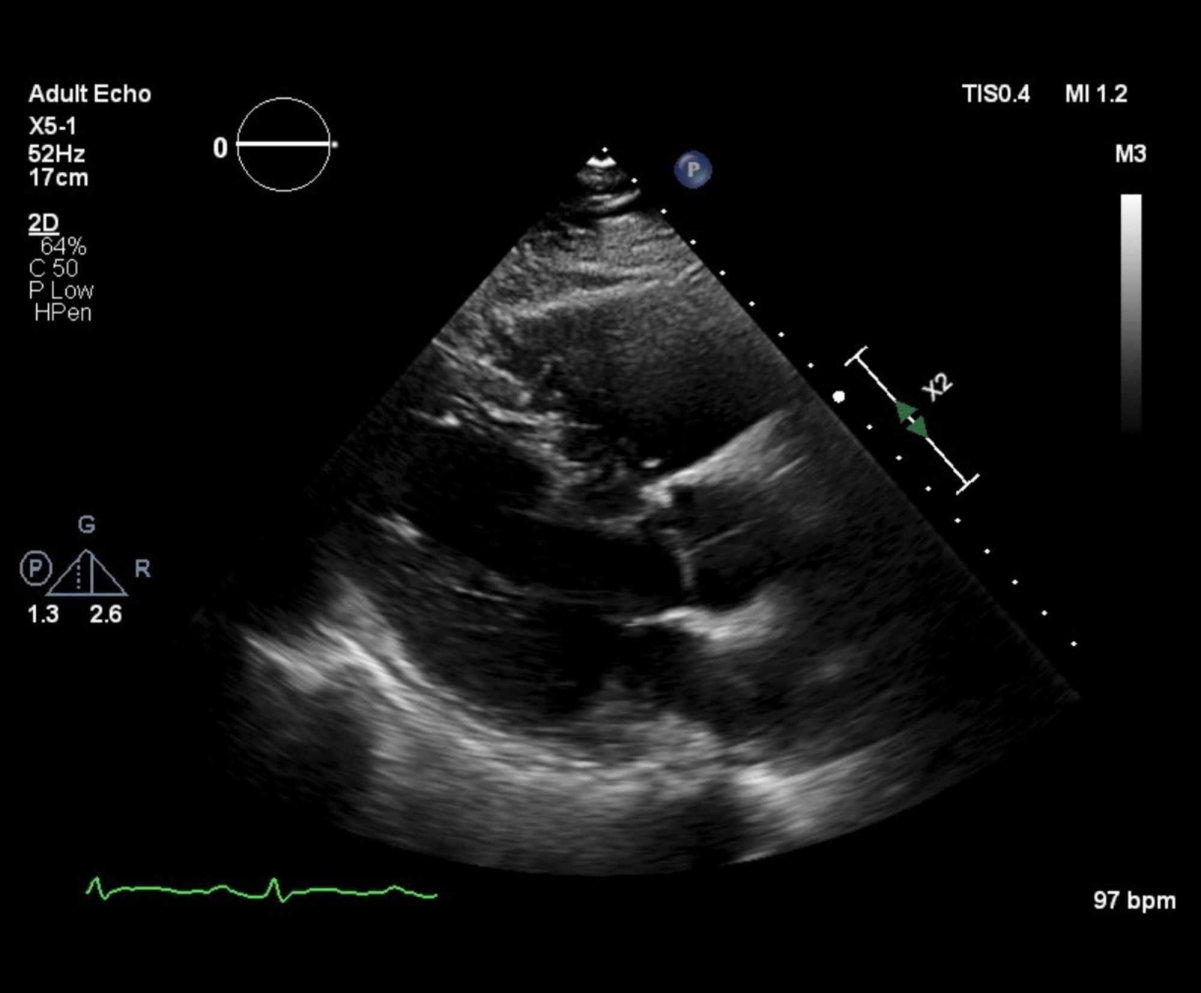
Parasternal long axis view on transthoracic echocardiogram (TTE) at one month post discharge Parasternal long axis view of TTE demonstrates resolution of pericardial effusion one month following discharge.
